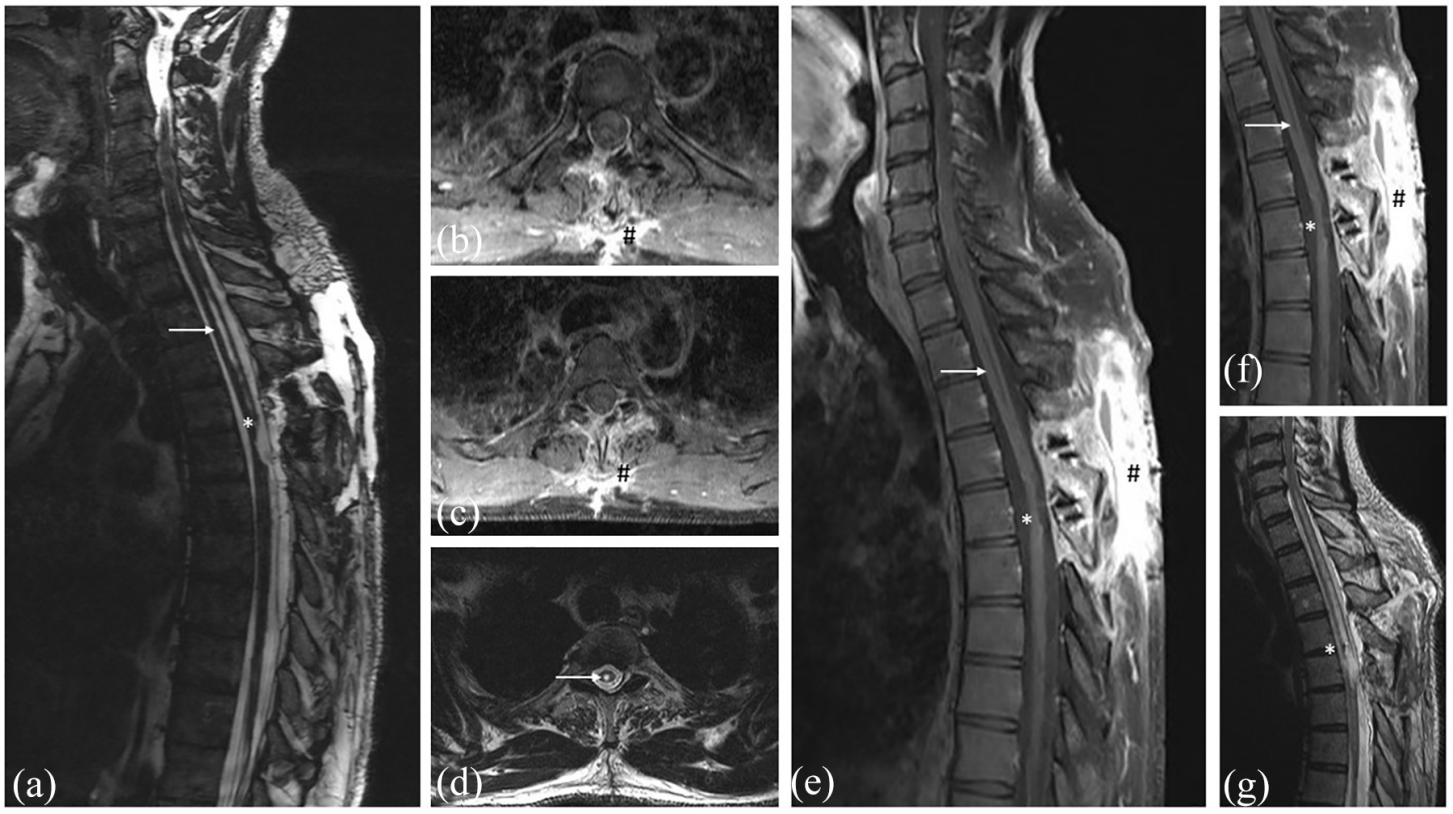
Early postoperative T2-weighted MRI without contrast (a + d) and T1-weighted MRI with contrast (b + c) 24 h after surgery with completely removed ependymoma. T1-weighted MRI with contrast (e + f) 6 months after surgery showing no tumor recurrence (*) despite the normal contrast enhancement at the dorsal approach (#). T2-weighted MRI without contrast showing the postoperative changes of the spinal cord (*) and the smaller edema of the spinal cord (→) (g).
